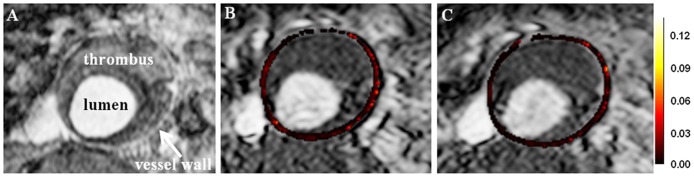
Scan-rescan reproducibility of *K^trans^*. (A) T1weighted anatomical image showing AAA with maximal diameter of 5.0 cm. (B and C) DCE-MRI images with overlay of parametric *K^trans^* maps from the same patient obtained from scans performed one week apart. The Patlak model was used. The left lateral AAA vessel wall exhibits higher *K^trans^* on both examinations. *K^trans^* maps are color-coded from 0 to 0.12 min^−1^.
